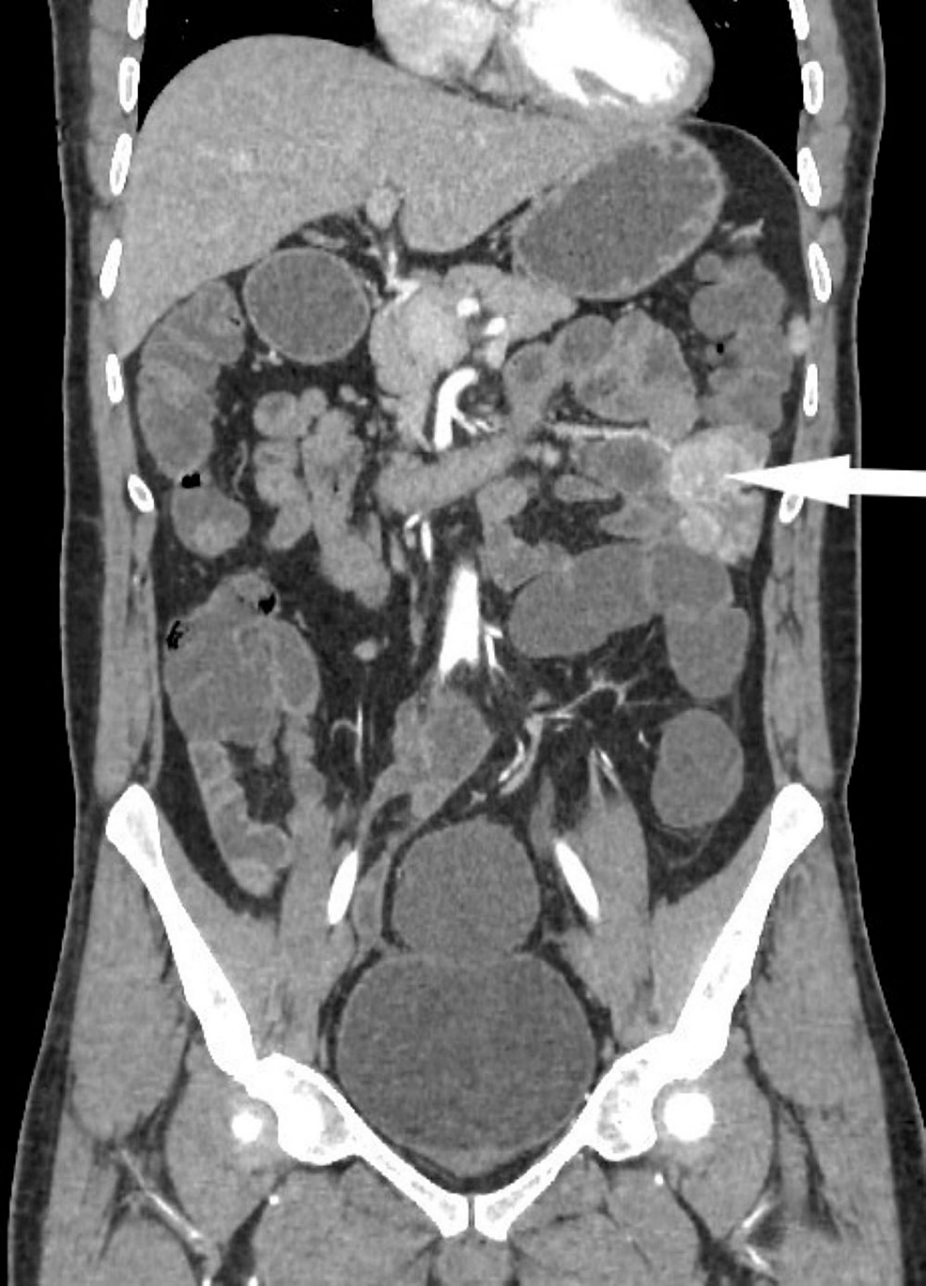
The entero-CT showing the jejunal mass
